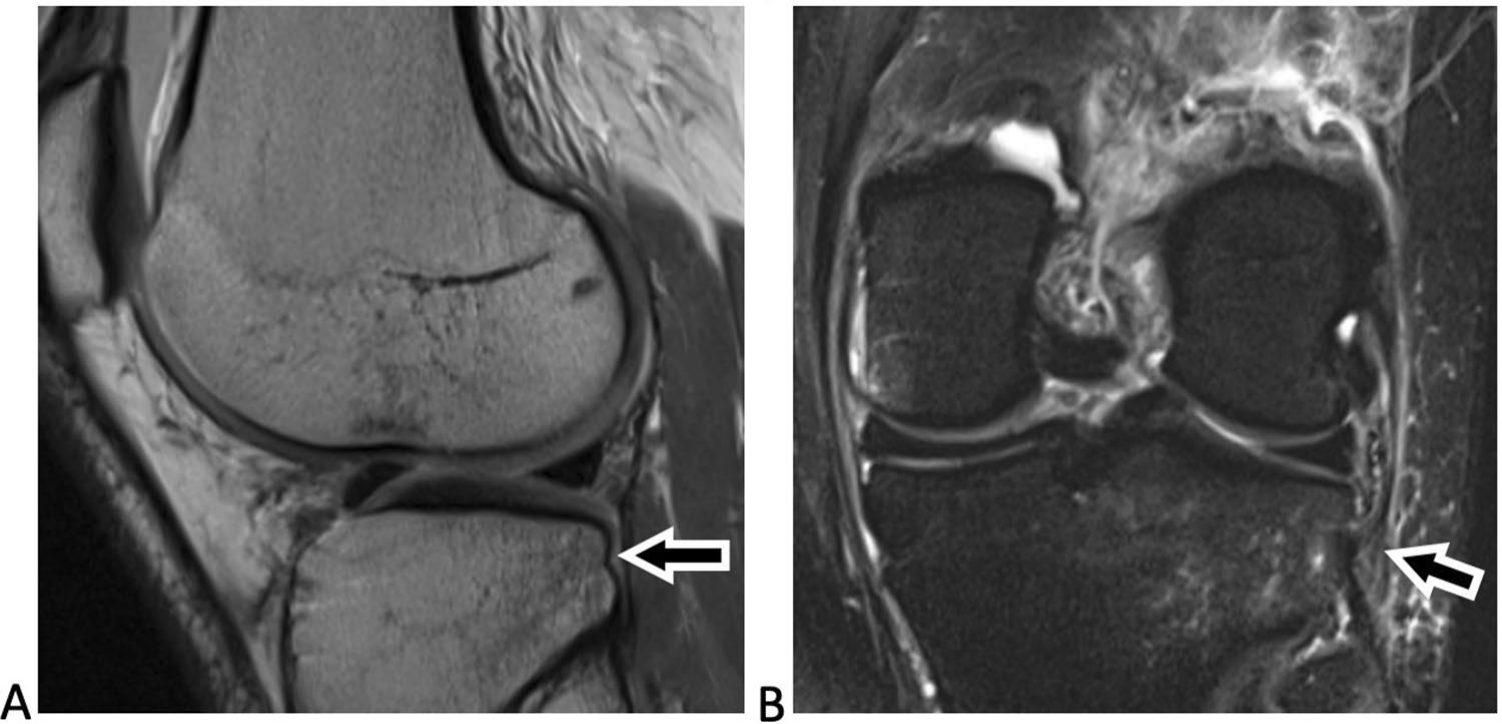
Magnetic resonance imaging of the left knee joint of a 24-year-old patient after ACL injury. The sagittal proton-density weighted image (A) shows a posterolateral tibial plateau fracture not involving the articular surface with a small cortical buckle (arrow in A), corresponding to a Type I fracture. The coronal T2-weighted fat-suppressed image (B) shows hyperintense signal alteration with intact fibres of the distal anterolateral complex (arrow in B), consistent with a low-grade injury
